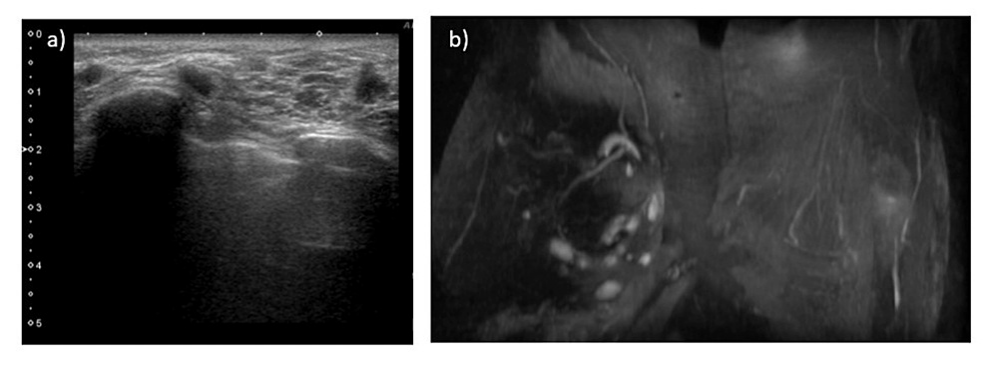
Imaging findings of local recurrences. Ultrasound examination (a) and MRI (b) of local recurrence under the skin. Ultrasonography shows multiple irregular, haloed masses, the largest of which is 12 mm in diameter, subcutaneously on the caudal side of the skin flap. MRI shows multiple subcutaneous irregular masses with contrast effect from the early phase.
